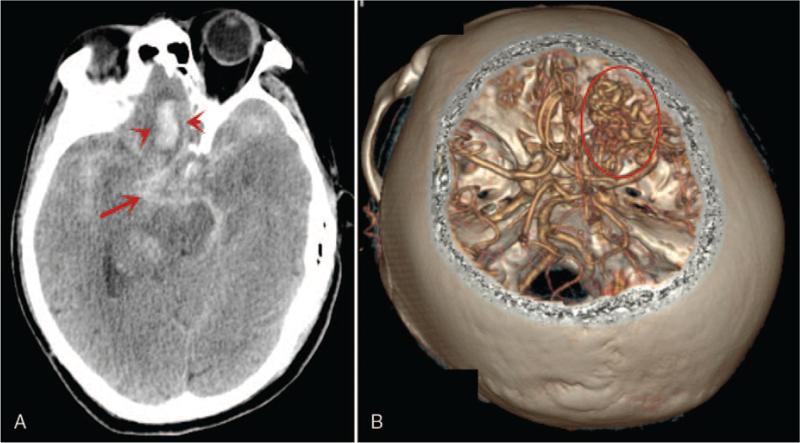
A and B: Are computed tomographic (CT) scan and computed tomographic angiogram (CTA). A: Subarachnoid hemorrhage (SAH) and hematoma in the right frontal lobe. B: Right frontal arteriovenous malformations (AVMs) with 3 intracranial aneurysms (IAs). Red circle = AVM; Red long arrow = SAH; red short arrows = hematoma in right frontal lobe.
